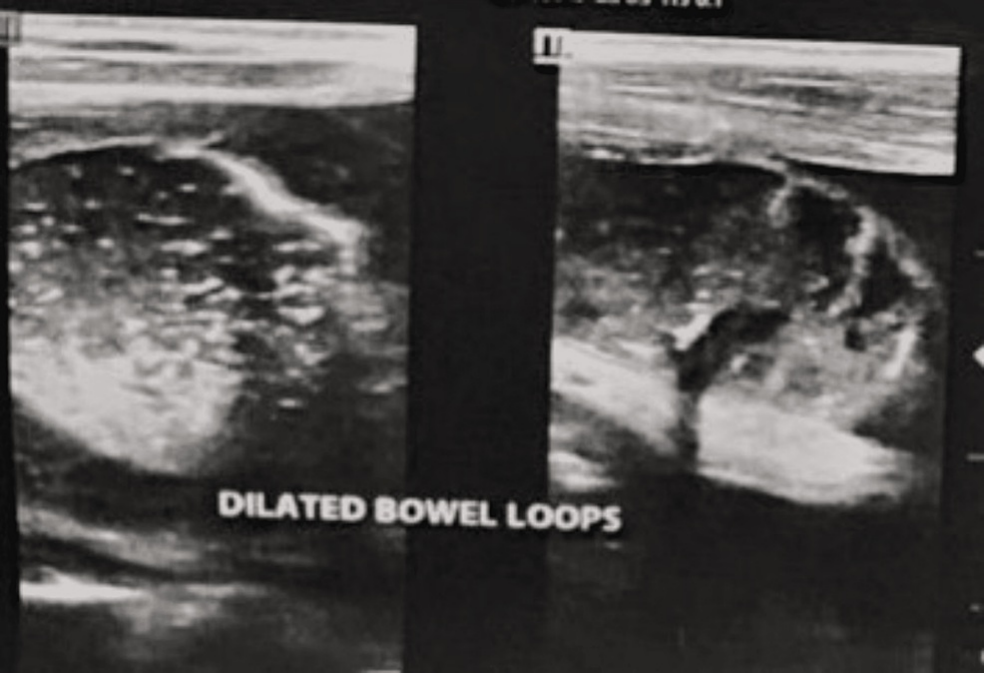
The patient's abdominal ultrasound demonstrated dilated loops of bowel with reduced peristalsis.
